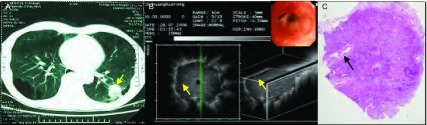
男，71岁，咳嗽胸痛1个月，低分化腺癌。A：CT见左肺下叶背段31 mm×22 mm圆形病灶；B：支气管镜下未见异常，支气管内超声于左下叶背段见病灶呈低回声，边界清晰，内部回声均匀，无支气管充气征，可见内部支气管狭窄（箭头所指）；C：病灶内可见支气管狭窄（箭头所指），瘤细胞大部分排列成实性巢状，分布密集，部分呈腺管样分化，灶性坏死，间质纤维增生，无支气管肺泡充气相（HE，×10）。 Male, 71 years old, cough and thoracic pain for 1 month, poorly differentiated adenocarcinoma. A: CT shows round lesion of 31 mm×22 mm at the apical segment of left lower lobe; B: No abnormality was found by bronchoscope, low echogenicity lesion was found at the apical segment of left lower lobe by endobronchial ultrasound probe, contour was clear, internal echo was homogeneous, no air bronchogram, bronchostenosis can be seen (at the arrow tip); C: Bronchostenosis can be seen in the lesion (at the arrow tip), tumor cells were mostly arranged as nestlike, intensively distributed, some of them showed crypt shaped differentiation, focal necrosis, and interstitial fibroplasias, no sign of air bronchogram (HE, ×10).
